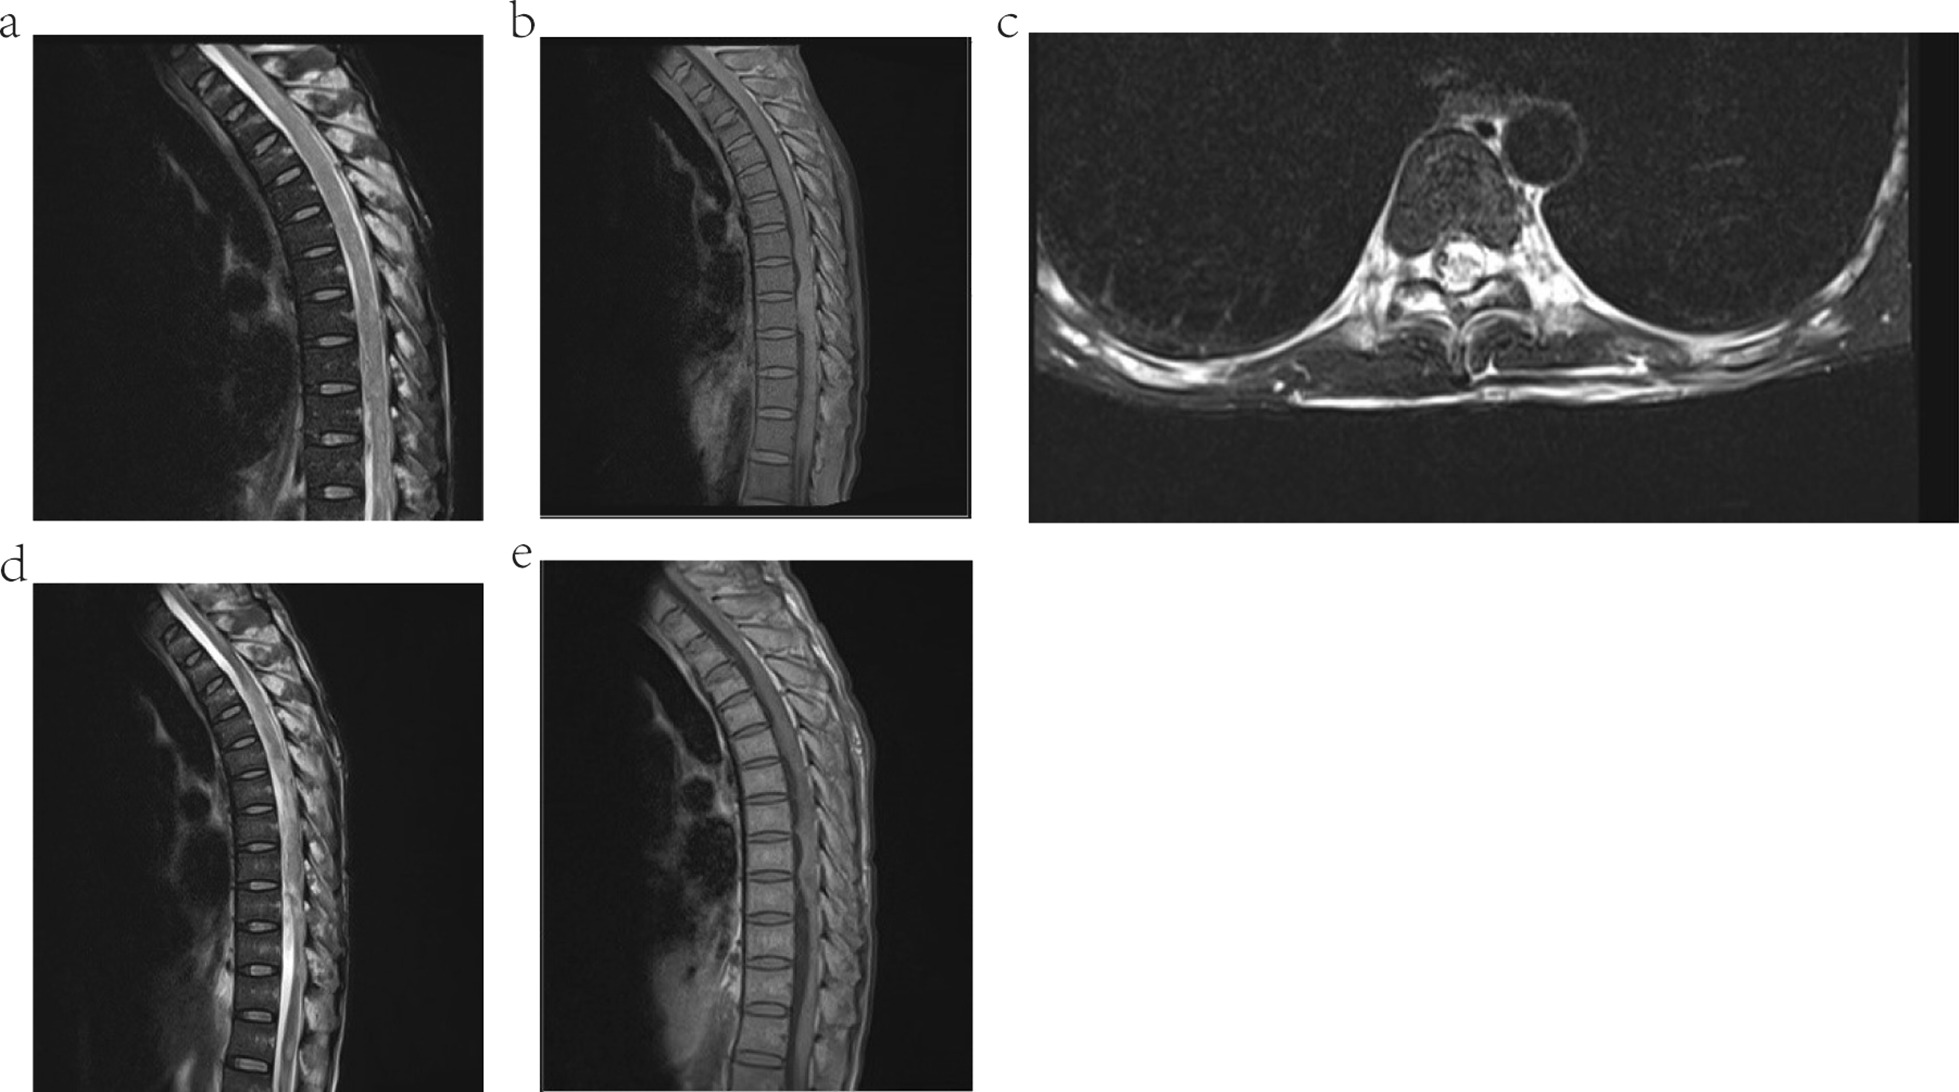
Spinal cord MRI and the mNGS result of the patient. a and d were the spinal cord segmental MRI T2-weighted image of the patient, b and e were the spinal cord segmental MRI T1-weighted image of the patient, c was the axial spinal cord segmental MRI T2-weighted image of the patient. a–c were recorded on February 17th, 2020. a–c showed that the thoracic spinal cord was irregular in shape with local enlargement and spotted and patchy hypersignal, which indicated edema or infection. d, e were recorded on April 3rd, 2020. Compared to a, b the edema spinal cord was reduced after treatment
